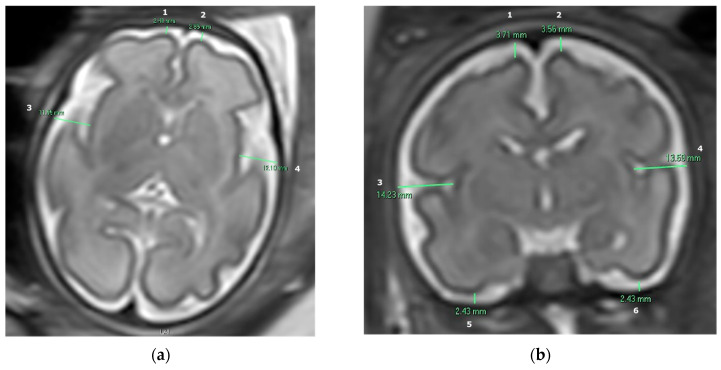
T2-weighted fetal brain MR image demonstrating the measurements of the subarachnoid space in millimeters: (a) Axial plane: (1) Right frontal gyrus. (2) Left frontal gyrus. (3) Right insula gyrus. (4) Left insula gyrus. (b) Coronal plane: (1) Right frontal gyrus. (2) Left frontal gyrus. (3) Right insula gyrus. (4) Left insula gyrus. (5) Right inferior temporal gyrus. (6) Left inferior temporal gyrus.
